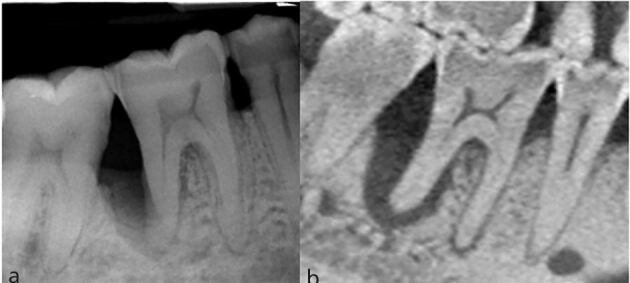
a, b) PA and sagittal slice CBCT showing J-shaped radiolucency associated with the distal root of tooth 46. The distal root in fact had a root groove, rather than a VRFRoot fractures (to some extent)
